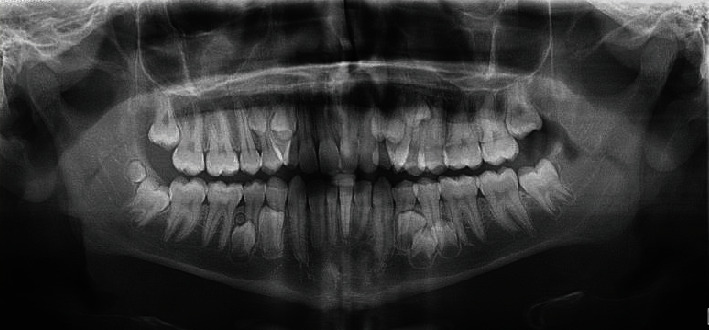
The original routine panoramic radiograph, showing supernumerary teeth.
